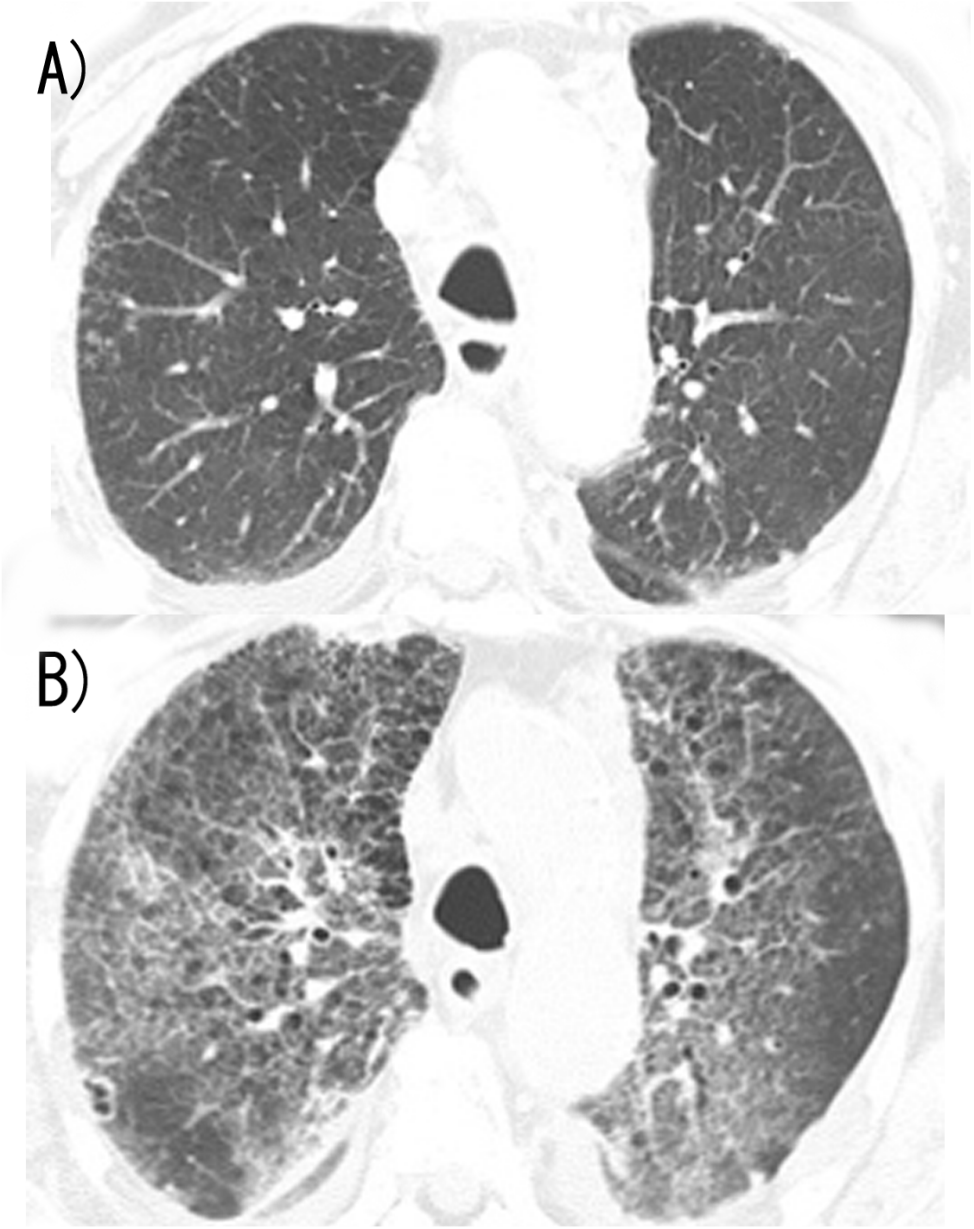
Chest computed tomography (CT) image of case no. 6. A) CT image obtained 7 days before irradiation to the thoracic spine showing a mild sub-pleural interstitial shadow and emphysema. B) CT image showing bilateral extended ground-glass abnormality superimposed on the pretreatment interstitial shadow.
